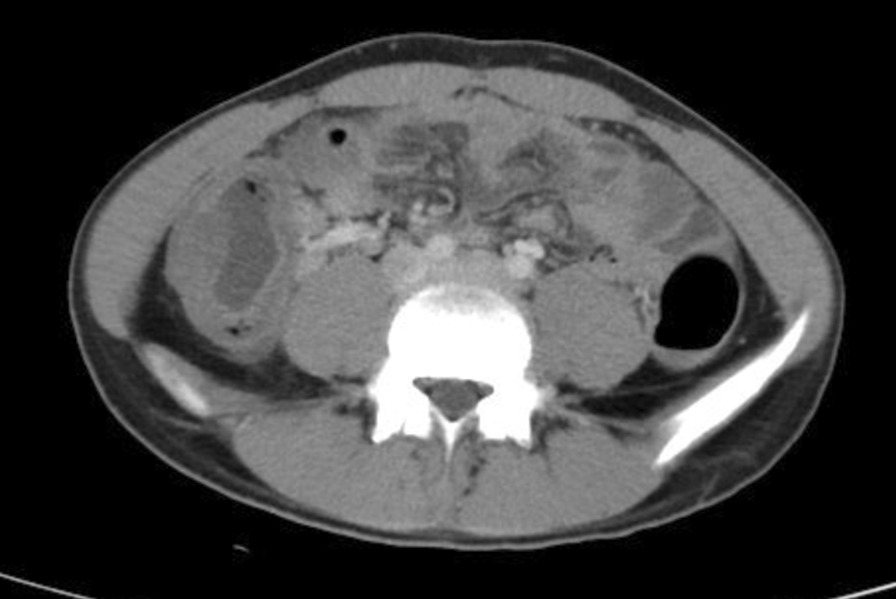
Non-contrast-enhanced computed tomography revealed acute enterocolitis consisting of moderately distended, thickened, fluid-filled loops of the ascending colon and transverse colon, and intramural air noted at the ascending colon
